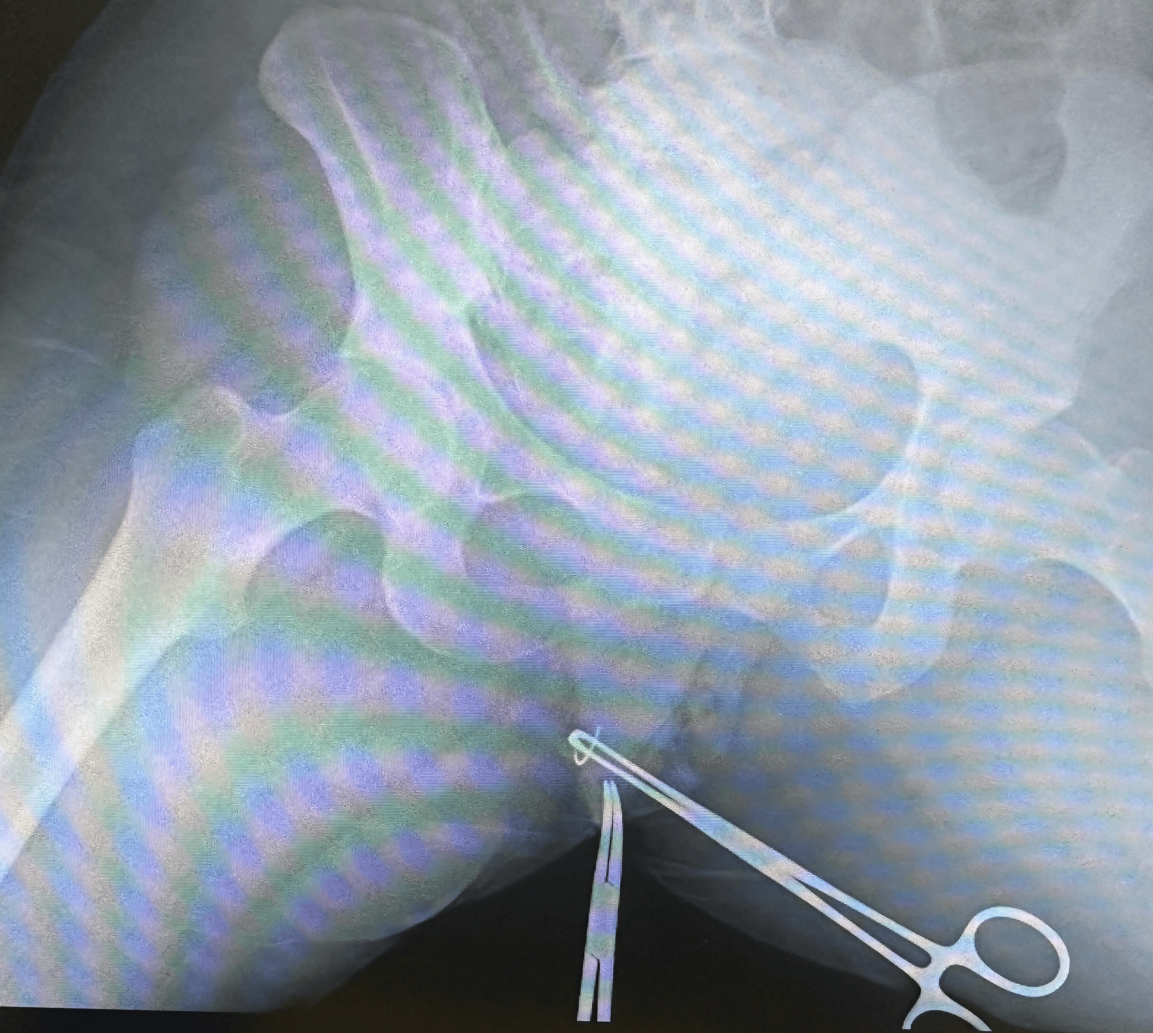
Intraoperative radiography-guided localization using metallic instruments. Intraoperative radiography-guided dissection was performed using a second metallic instrument positioned near the suspected target area to facilitate accurate localization of the retained needle fragment.
